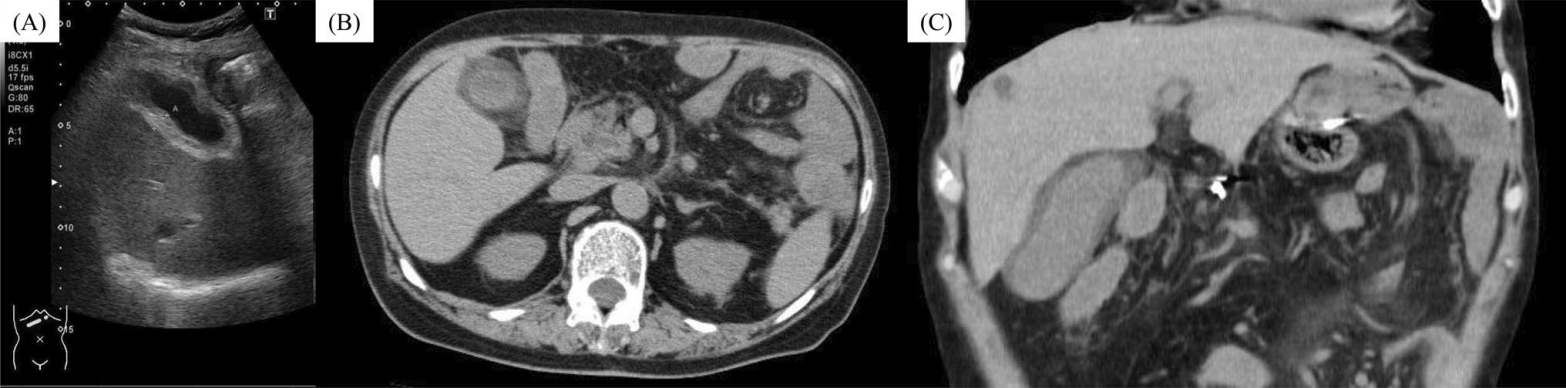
Imaging findings at the primary care physician at the onset of right upper abdominal pain. A Abdominal US showing gallbladder wall thickening of approximately 9 mm. B, C CT demonstrating diffuse thickening of the gallbladder wall without increased attenuation of the surrounding fat tissue
